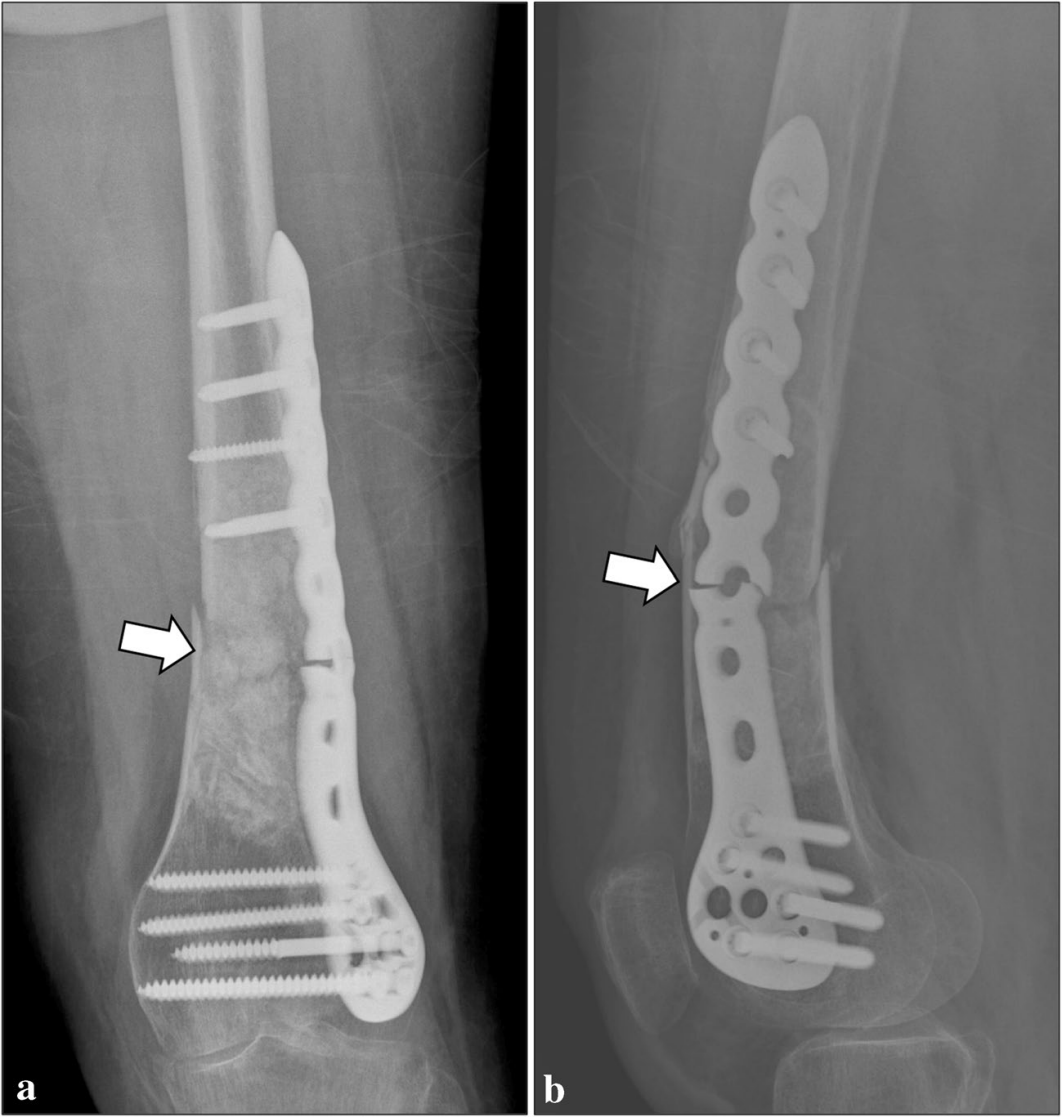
Post-operative bone fracture and plate rupture in a 55-year-old woman. Bone fracture and plate rupture (arrows) occurred 17 months after curettage of an ACT of the distal femur, bone grafting and internal fixation, as shown on frontal view (a) and lateral view (b) radiographs. Fixation surgery was performed using a longer plate and progressive healing occurred thereafter (not shown)
